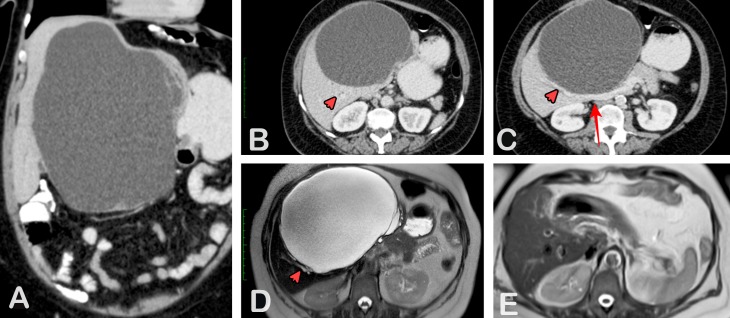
The preoperative contrast enhanced the by the computed tomography and magnetic resonance imaging. a: coronal reconstruction, b and c: axial slices and d: the T2W magnetic resonance imaging, demonstrates a large cystic mass with the maximum diameter of 21.5 cm. There are a few thin internal septations that show mild contrast enhancement but no focal wall thickening or other solid components in the mass. The lesion occupies II, III, IV, V ,and VIII hepatic segments and displaces intrahepatic vessels with no sign of vascular invasion (red arrow: main portal vein). The intrahepatic ducts at the periphery of the mass are slightly dilated due to the mass effect (red arrowheads), e: the T2W magnetic resonance imaging after the lesion excision.
